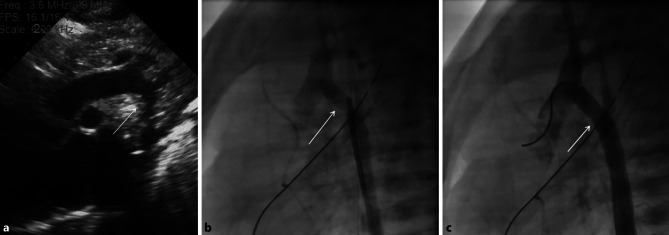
a Echocardiographic image of the aortic arch. Note the thrombus presenting as an irregular shape at the inner curvature, which is echodense. b Hand injection into the transverse arch. *Arrow* Less contrast in the distal arch representing thrombus (also confirmed on echocardiography). c Stent in the transverse arch, no residual thrombus visible
